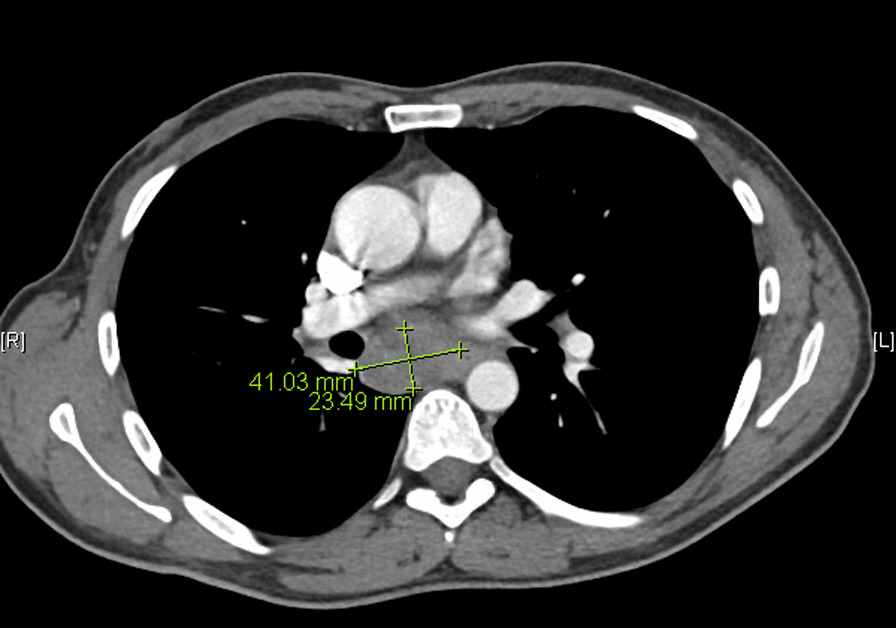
CT chest with contrast showing mediastinal mass invading esophageal wall
